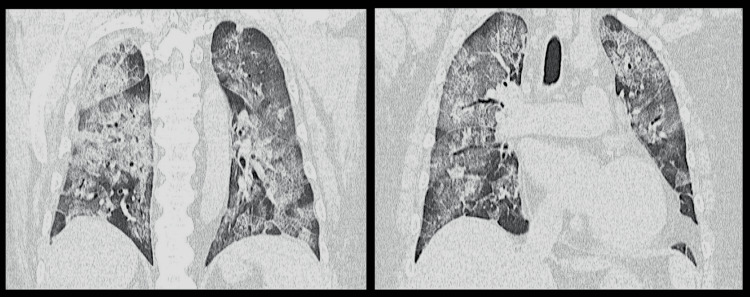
CT scan showing ground-glass shadows throughout both lung fields CT: Computed tomography
